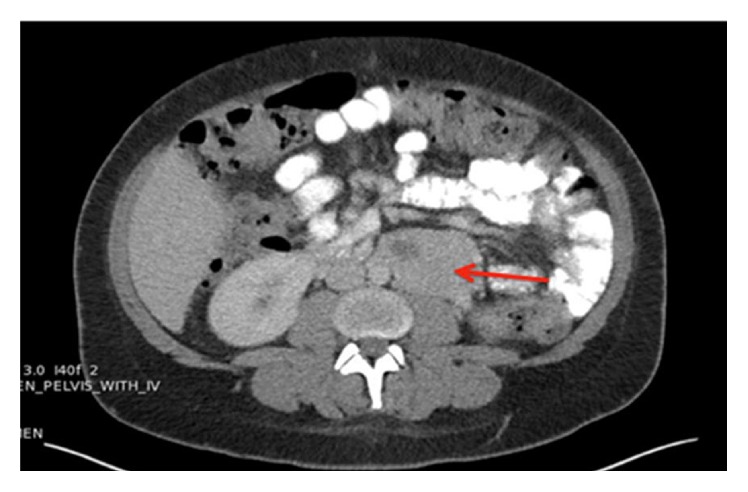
CT abdomen/pelvis demonstrating a left para-aortic mass measuring approximately 4.7 × 6.4 cm.
